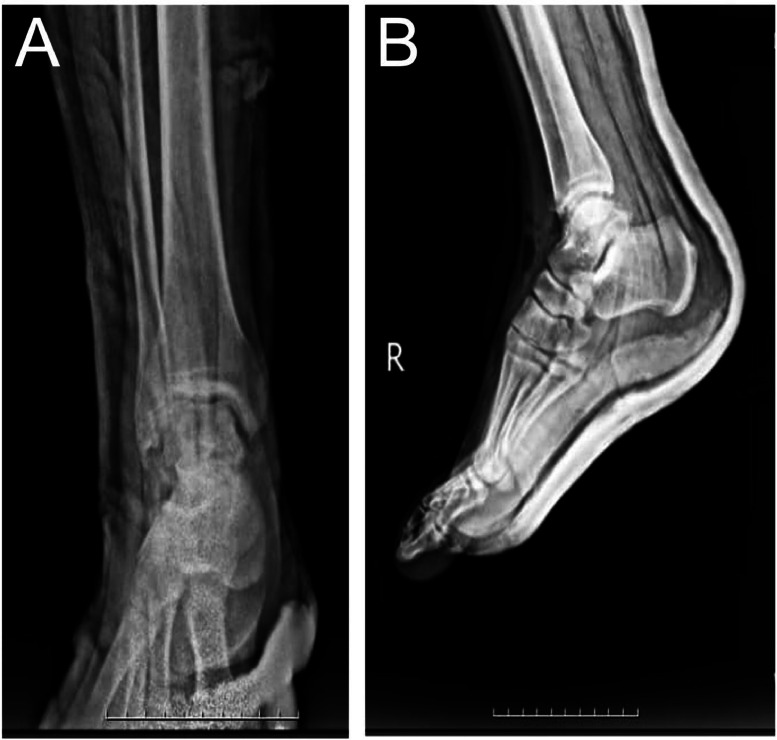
Postoperative plain radiographs of the right foot and ankle 1 week after surgical removal of loose bodies. No residual calcified synovial chondromatosis lesions are observed. (A) Anteroposterior (AP) view, (B) Lateral view. The images demonstrate a clear joint space without evidence of remaining intra-articular fragments.
